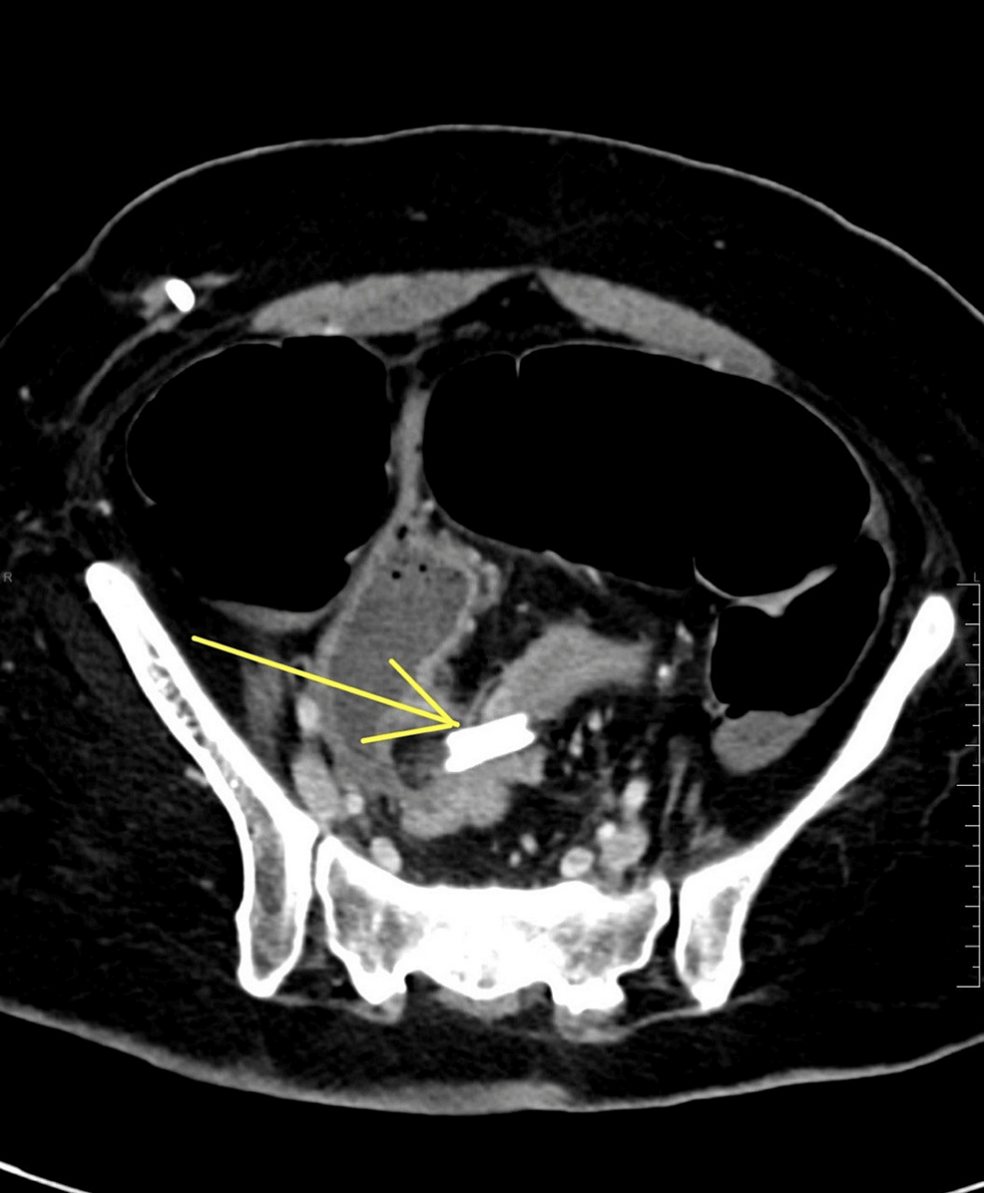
Computed tomography of the abdomen showing a closed-loop bowel obstruction at the level of the sigmoid colon caused by the coiling of the ventriculoperitoneal shunt catheter
